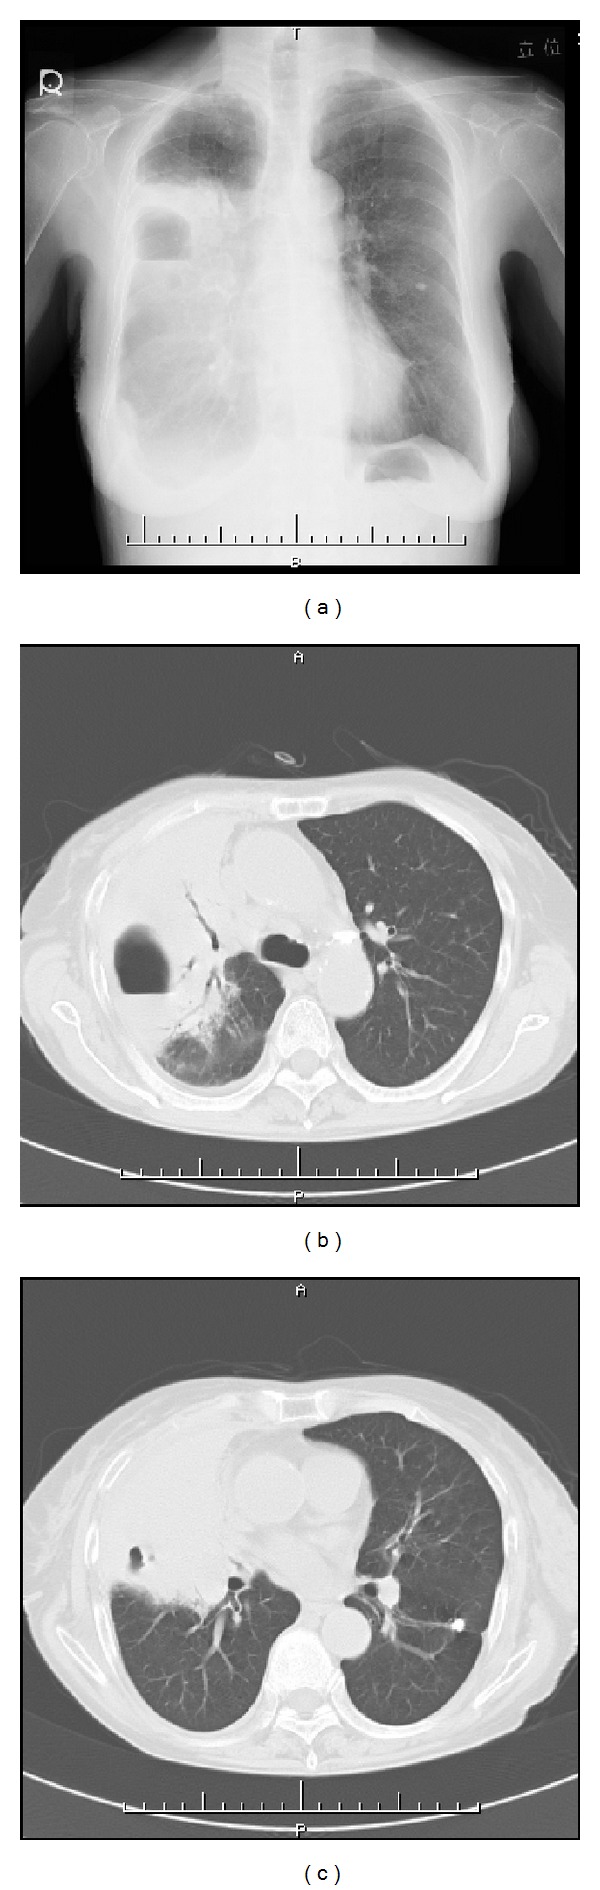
Chest X-ray (a) and CT ((b) and (c)) six years before the patient was referred to our hospital. CT revealed a lung abscess in the S2 field of the right lung and consolidation around this abscess.
